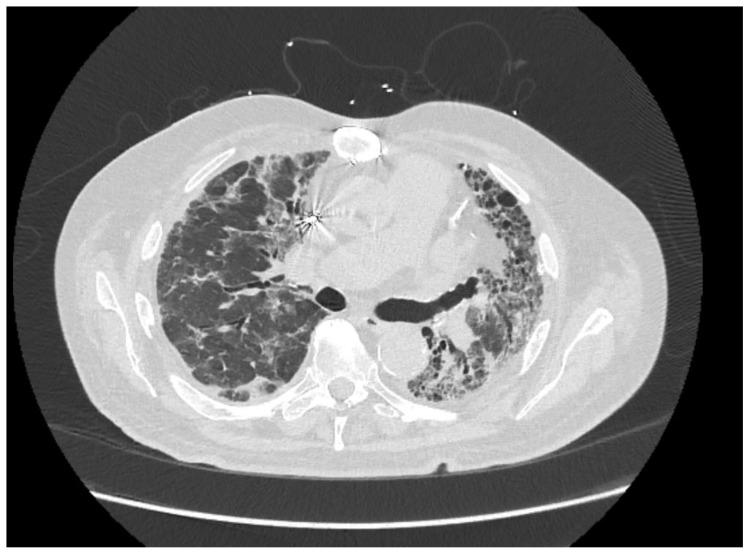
Axial view of chest computed tomography (CT) at the subcarinal level, showing advanced left lung fibrosis (with traction bronchiectasis and reticulation) and more acute right lung inflammation (ground glass opacities mixed with traction bronchiectasis).
